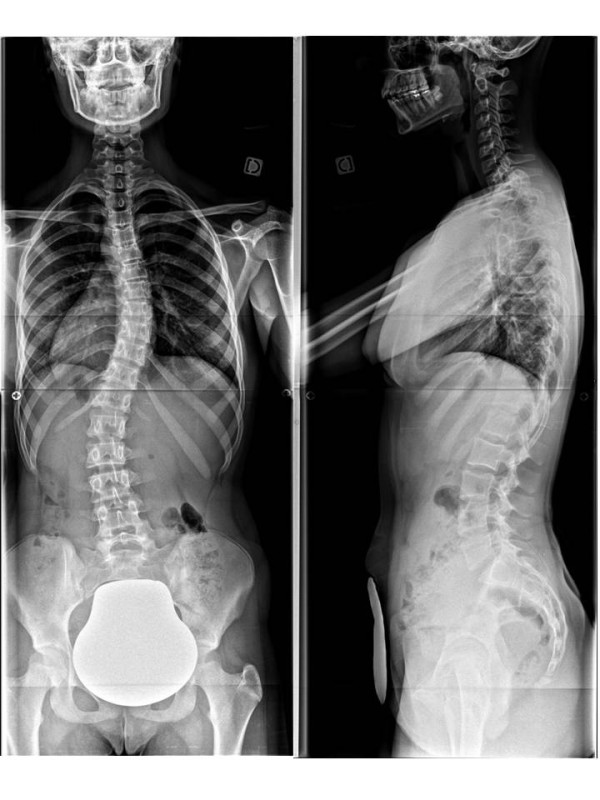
Face and profile x-raysin CMCR.
